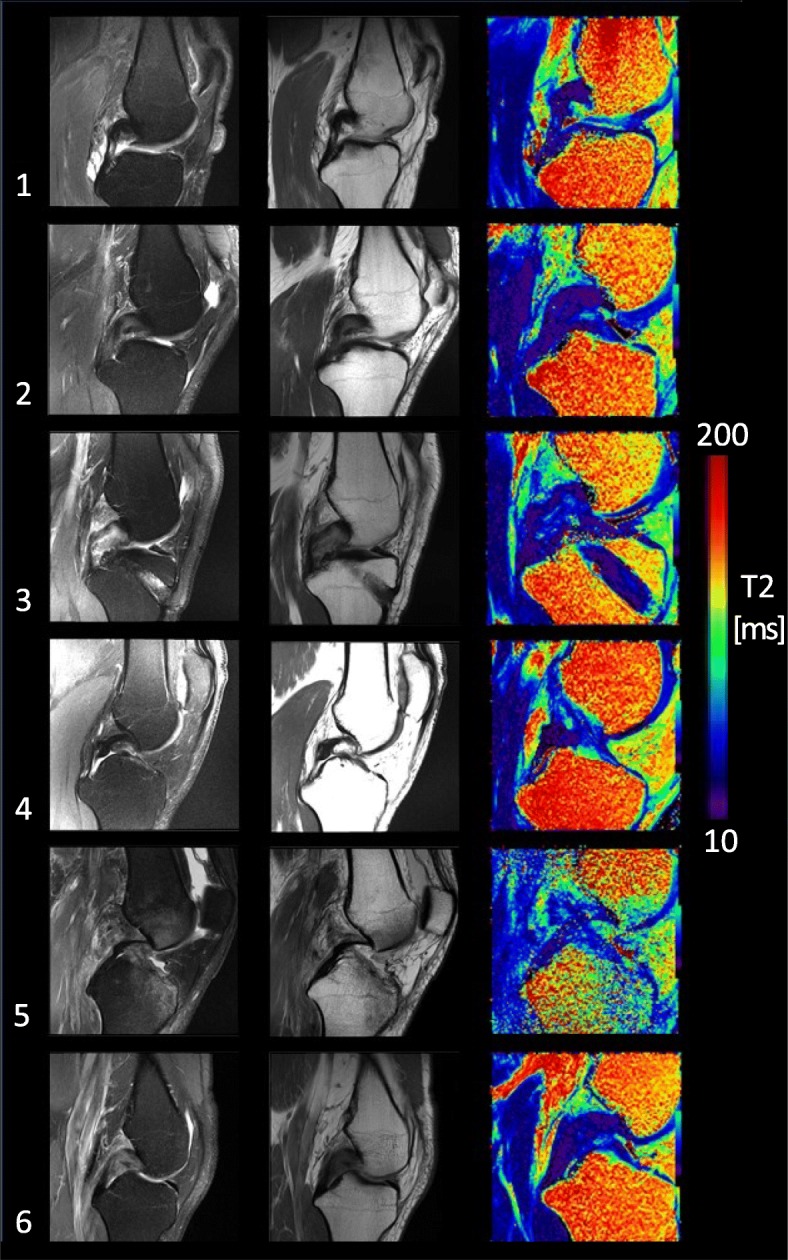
Example MR images in the six individual patients with PCL insufficiency showing a continuous ligament. Images show the closest sagittal slice through the middle of the injured PCL for each of the following sequences: PD FS TSE, PD TSE and color-mapped T2 mapping image
